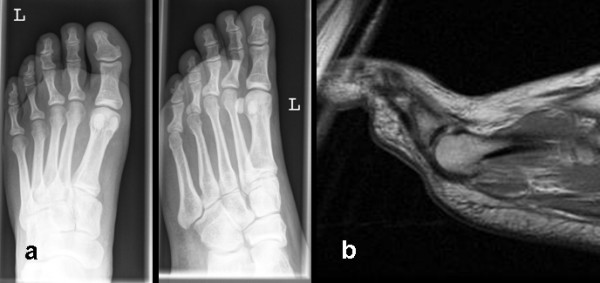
This figure shows anterioposterior and 3/4 (A) conventional radiographs and a T1 weighed MRI section in the sagittal plane (B) of the third toe of the left foot. A. There is a small fracture at the base of the proximal phalanx of the third toe. B. This MRI section shows an intra-osseous lesion, without expansion beyond the cortical border of the phalanx.
